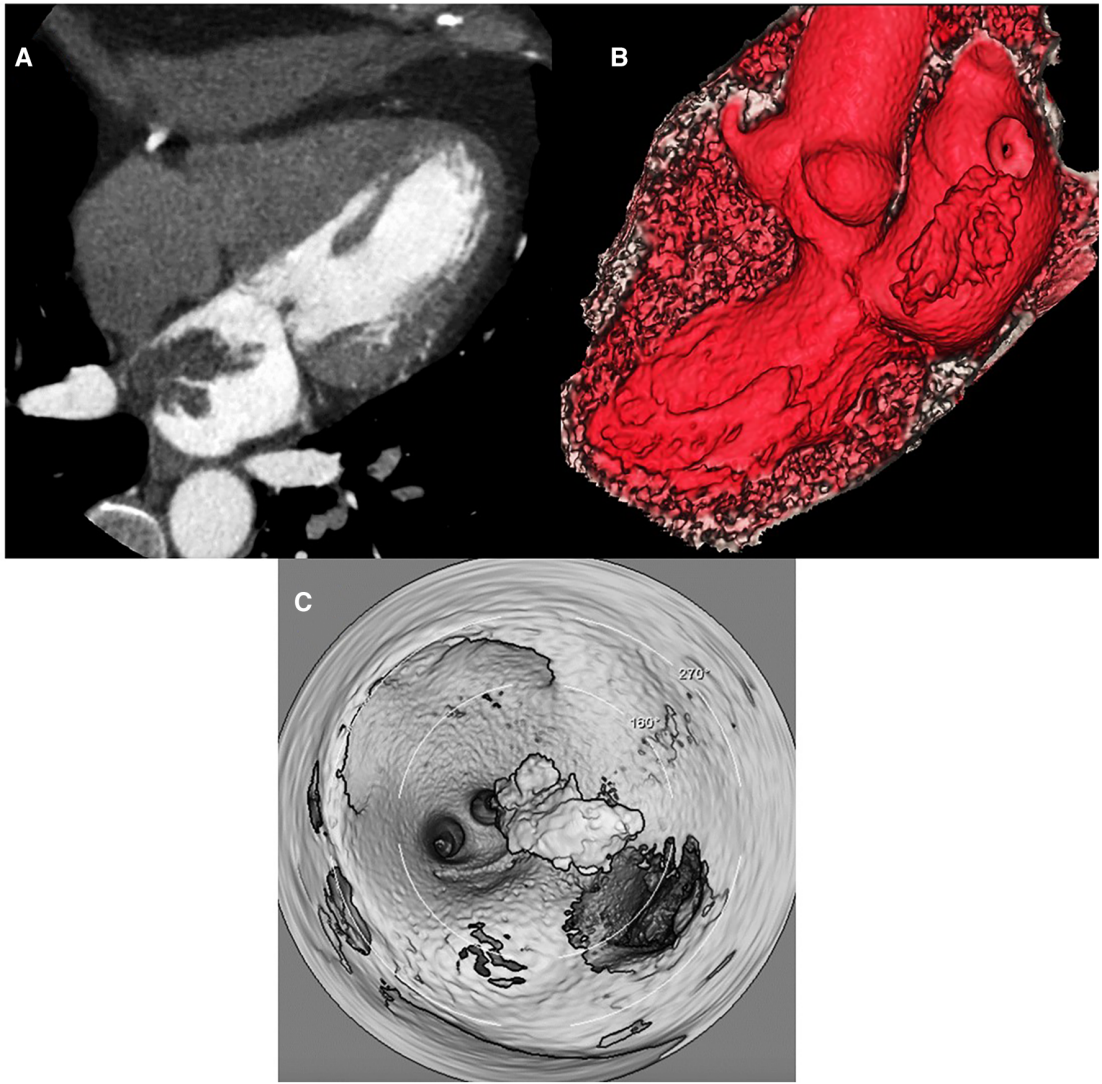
Cardiac computed tomography/3D-tools. (A) Cardiac contrast enhanced computed tomography horizontal long axis (4 – chambers) shows a lobulated, frond-like left atrial mass with villous borders (high risk of embolism), diameters of 39 × 39 × 20 mm, heterogeneous, isodense with punctate hypodense areas (clots), arising from the upper interatrial septum. (B) Oblique reformat volumen rendered 3D cardiac tomography demonstrated shows realistic anatomical relationships of left atrial mass near the ostium of the right inferior pulmonary vein. (C) Three-dimensional intracardiac navigation of 360 degrees revealed the dependence of the interatrial septum and the relationship with pulmonary veins of atrial mass.
